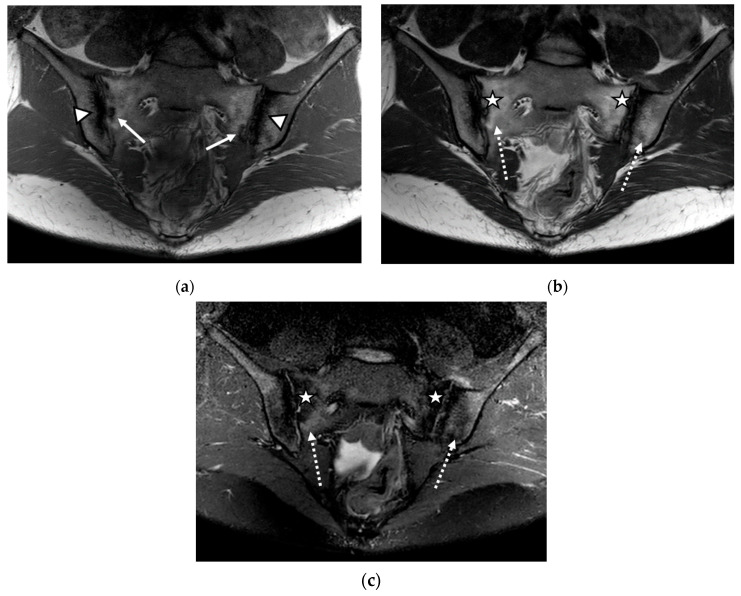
The magnetic resonance imaging (MRI) examination of the sacroiliac joints revealed narrowing of the sacroiliac joint spaces with bilateral marginal bone erosions (continuous arrow), bilateral subchondral sclerosis (arrowhead), and increased fat metaplasia (star), with minimal diffuse adjacent bone marrow edema (dotted arrow): (a) T1-weighted sequence; (b) T2-weighted sequence; (c) short tau inversion recovery (STIR) sequence.
